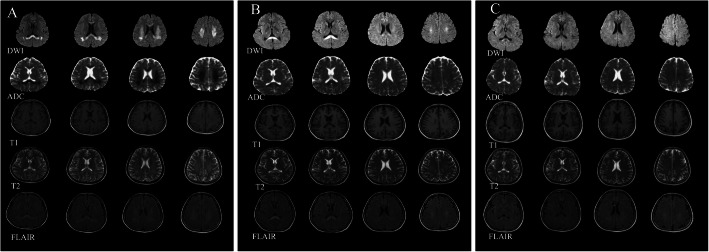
MRI findings during the initial emergency department visit and follow-up. a The MRI scan showed lesions in the corona radiata, bilateral centrum semiovale, bilateral posterior limb of the internal capsule and corpus callosum, extending laterally into the posterior deep white matter. b A subsequent MRI scan performed 9 days later showed an obvious limitation of the diffusion in the posterior corpus callosum, and there were significant improvements in the other areas that were previously damaged. c The MRI scan obtained at the 1-month follow-up visit showed that the abnormal signal alterations were mostly resolved
